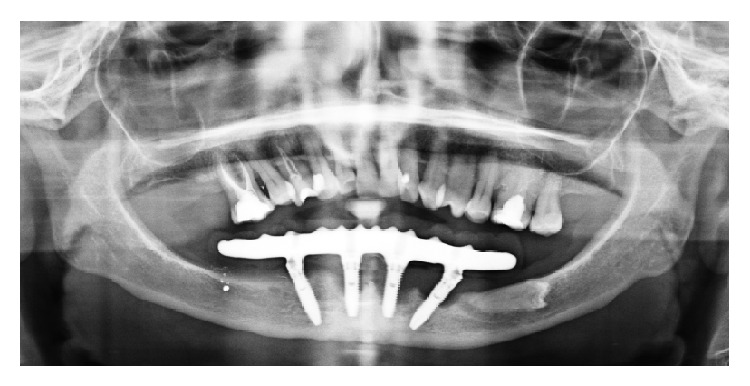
Orthopantomography of the patient after 84 months from surgery.
